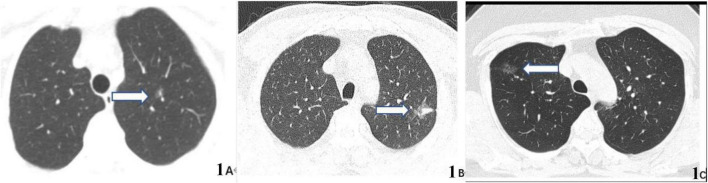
CT images of three typical lung nodule types correctly distinguished by our proposed model while wrongly determined by radiologist. (A) 59-year-old woman, CT scan of the chest showing a ground glass nodule in the upper lobe of the left lung with post-operative pathological result of adenocarcinoma *in situ* (AIS) (arrow). (B) 63-year-old man, CT scan of the chest showing a mixed ground glass nodule in the upper lobe of the left lung with post-operative pathological result of invasive adenocarcinoma (IA) (arrow). (C) 36-year-old man, CT scan of the chest showing a pure ground-glass nodule in the upper lobe of the right lung, which suggests an inflammatory lesion (arrows) owing to its disappearance on reexamination 3 months later.
